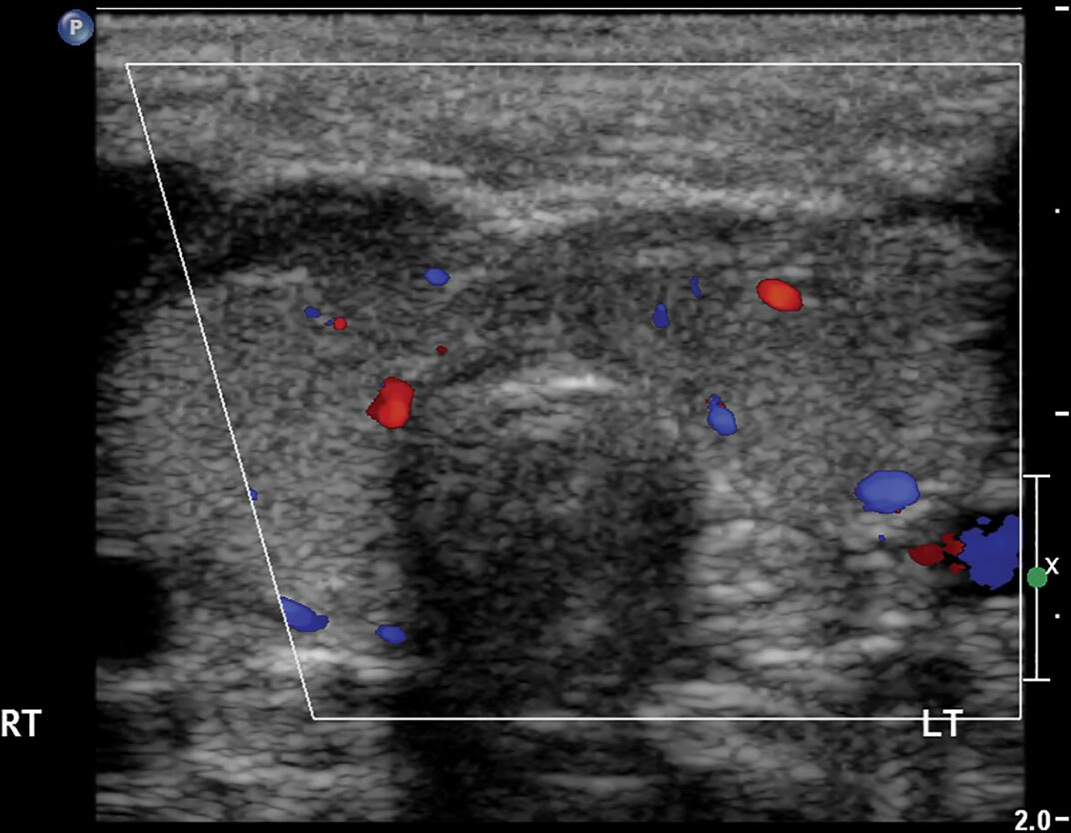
Ultrasound of infant with a variant of the brain–lung–thyroid syndrome with mutation adjacent to the *NKX2.1* gene (patient T6, Table 2). Subjective evaluation suggested a bulky gland attributable to a single, flattened, slightly curved anterior border but measured volume was small-normal at 0.94 ml. o PostScript
